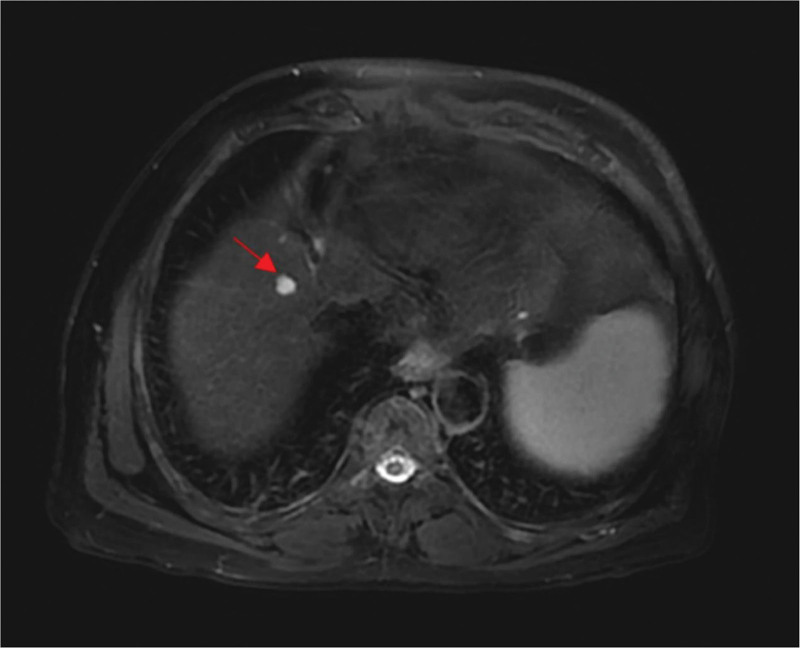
The contrast-enhanced liver MRI showing the patient’s HGDN. HGDN = high-grade dysplastic nodule, MRI = magnetic resonance imaging.
